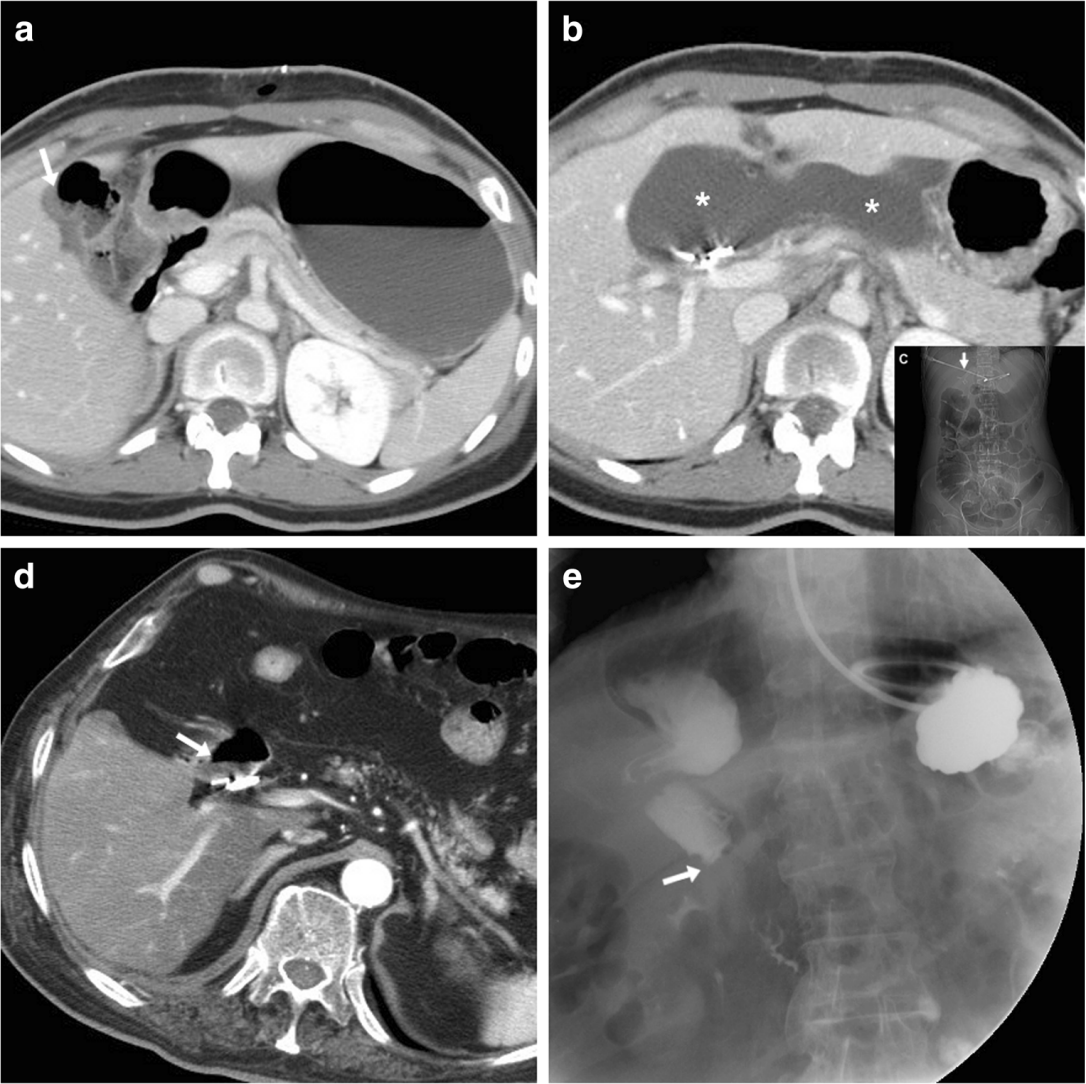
a–c Early postoperative CT after laparoscopic cholecystectomy showed gastric dilatation with stagnant fluid, minimal fluid in the gallbladder fossa (*arrow* in a) and a homogeneous water-attenuation collection (* in b) extending from the clips at the surgical site towards the lesser sac, consistent with biloma. Inset image c shows persistent gastrointestinal gaseous distension consistent with postoperative ileus, after percutaneous drainage (*thick arrow*) of biloma. d, e Shortly after laparoscopic cholecystectomy, CT showed clips adherent to the duodenal bulb (*arrow* in d), causing impaired transit of oral iodinated contrast during fluoroscopy (e)
